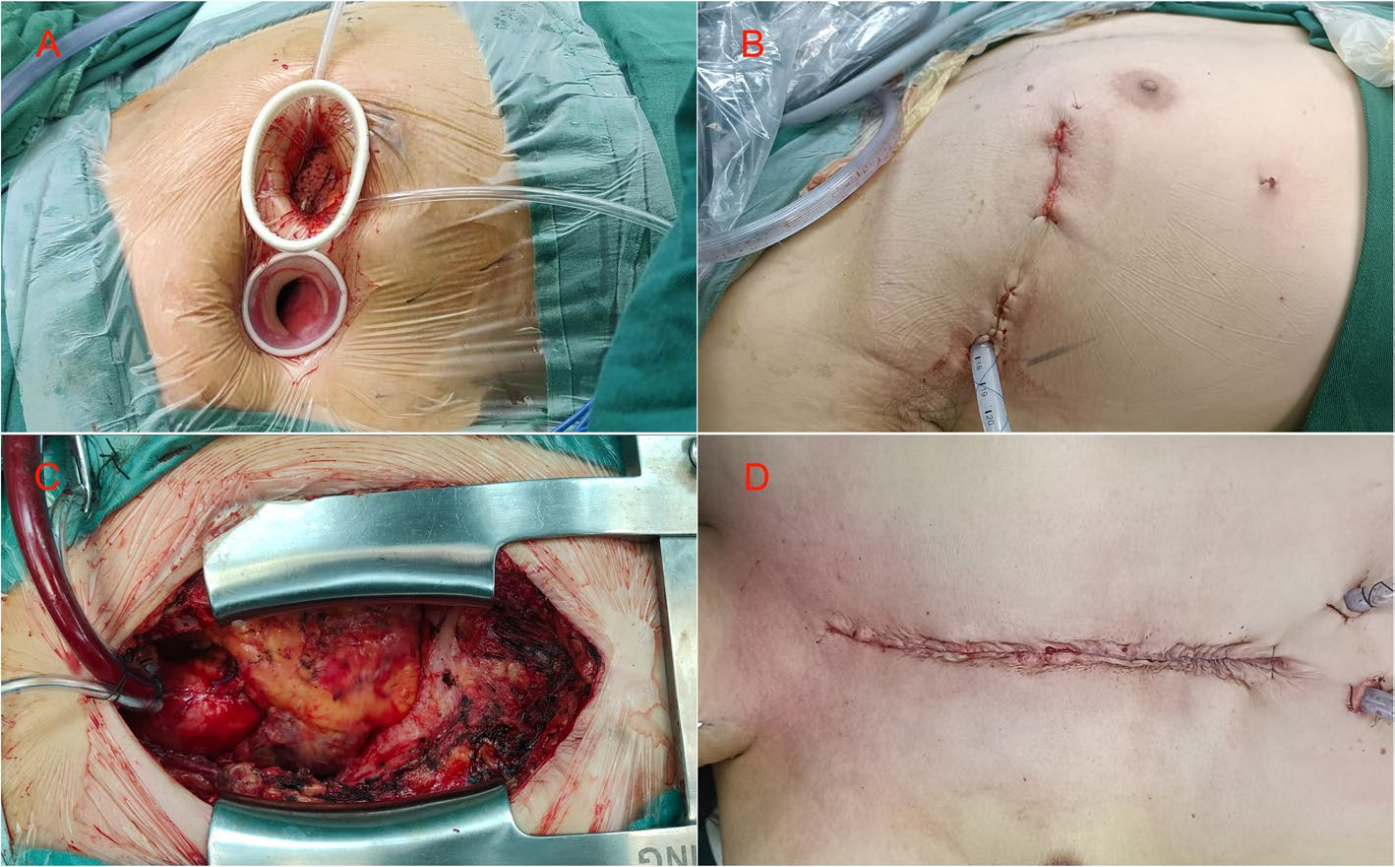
A comparison of the postoperative chest radiographs of a representative patient who underwent TTS (A, B) and a representative patient who underwent CFS (C, D), showing the difference of the surgical incisions and the chest tubes
